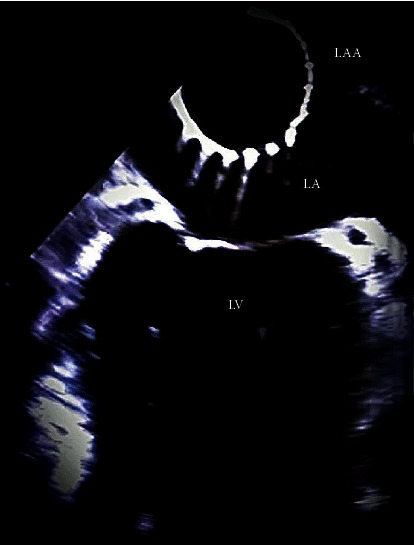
Mid-esophageal 45° TEE view showing the Watchman device floating freely in the left atrium after being dislodged from the left atrial appendage (TEE: transesophageal echocardiogram, LAA: left atrial appendage, LA: left atrium, and LV: left ventricle).
